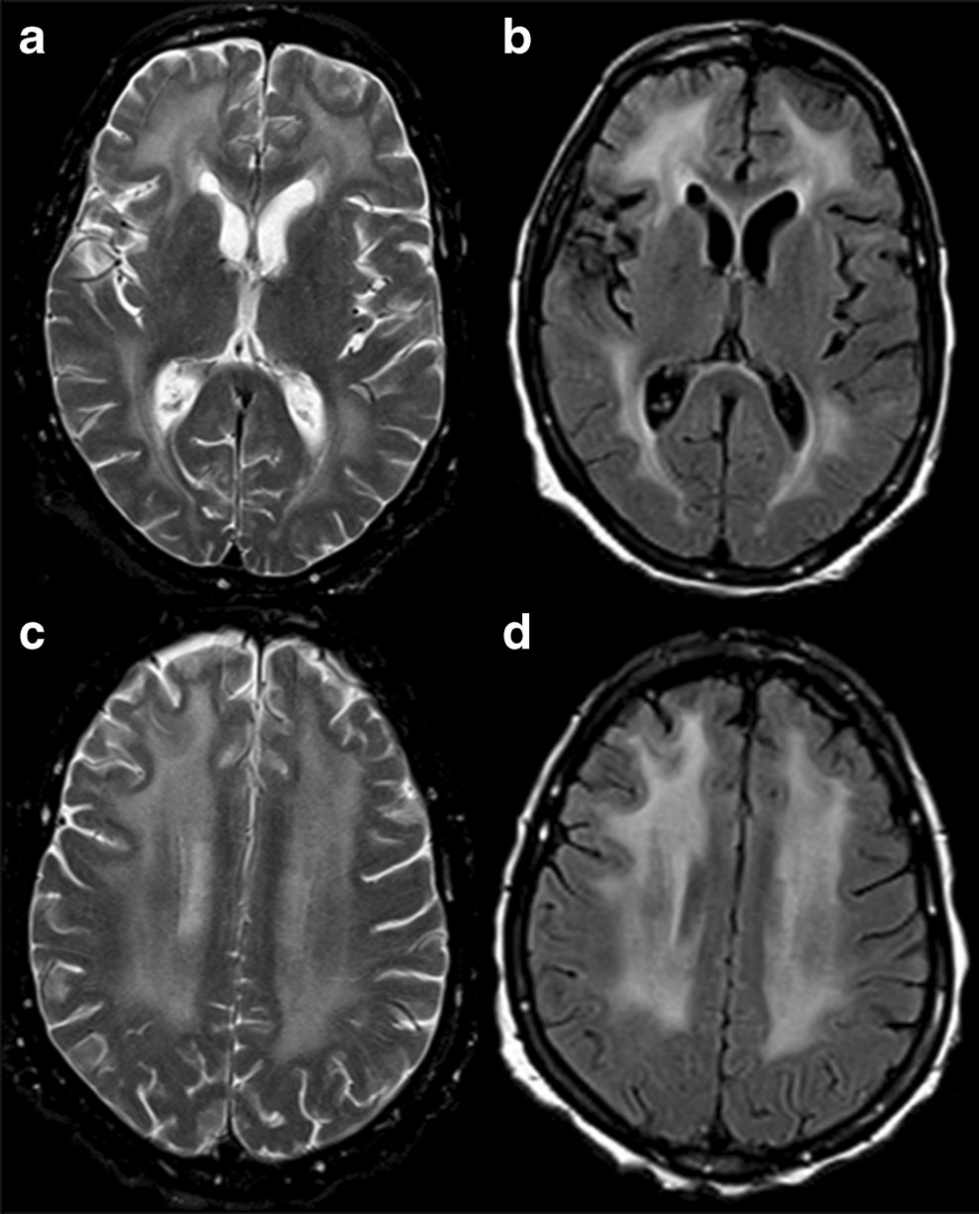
HIV-Associated Dementia (HAD). A 56-year-old female with a several-month history of generalized weakness, confusion and memory loss. a & b axial T2W and FLAIR images of the brain at the level of ventricles show diffuse symmetrical white matter hyperintensity and mild generalized atrophy. c & d axial T2W and FLAIR images at the level of centrum semiovale reveal similar diffuse symmetrical hyperintensity in the white matter
